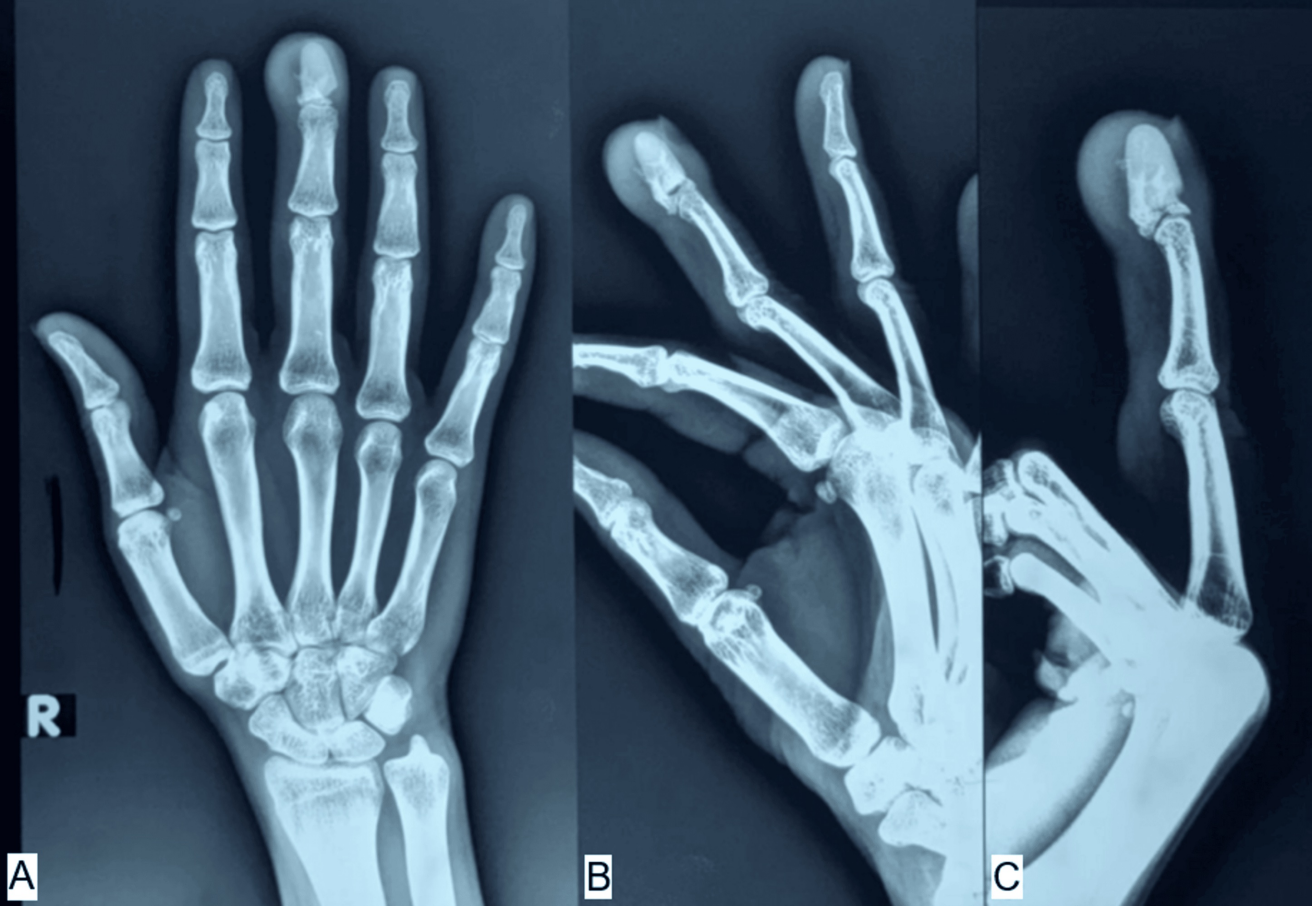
(A-C) X-ray shows an oblong-shaped radiopaque shadow of the cement bead at the tip of the middle finger
